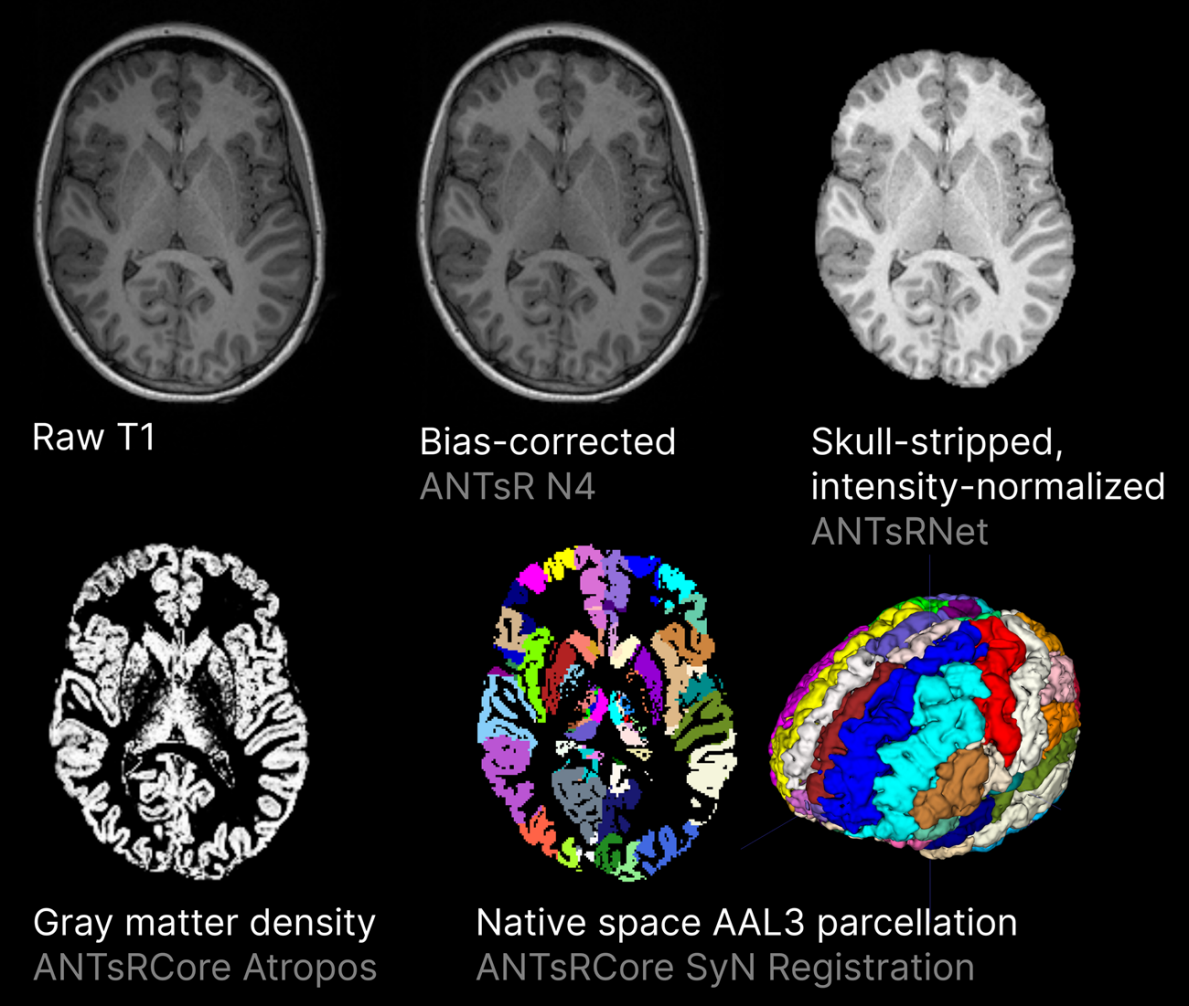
3D T1-weighted Image Volume Preprocessing.
